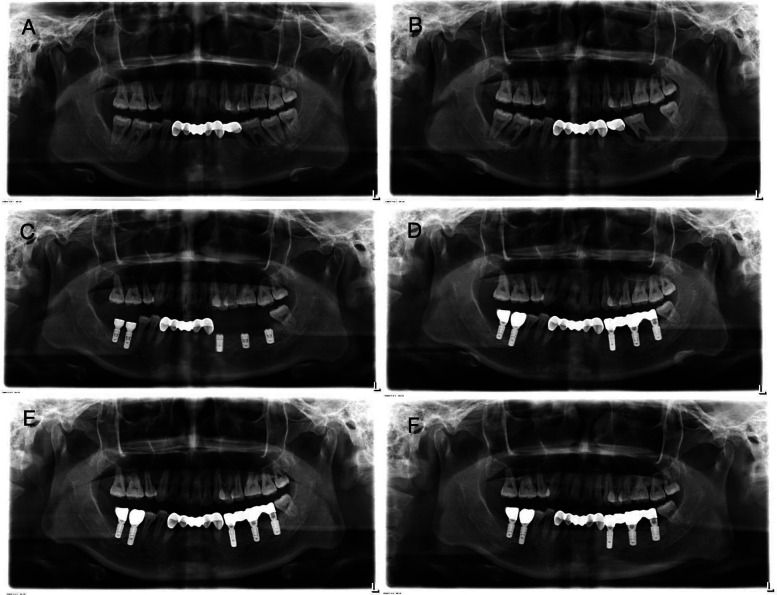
Panoramic view of case 1 patient. (A) First visit before pregnancy. The alveolar bone of tooth 36 was destroyed and absorbed to the apex, accompanied by the shadow of the root furcation. Significant radiolucent area surrounded the root apex of tooth 37. Bone resorption around tooth 46 was also present. (B) Four months after delivery. It revealed extensive radiolucency around the roots of teeth 36 and 46. (C) After implantation. (D) After implantation and restoration. (E) One month after implantation and restoration. (F) One year after implantation and restoration. The panoramic radiograph shows stable alveolar bone throughout the mouth.
